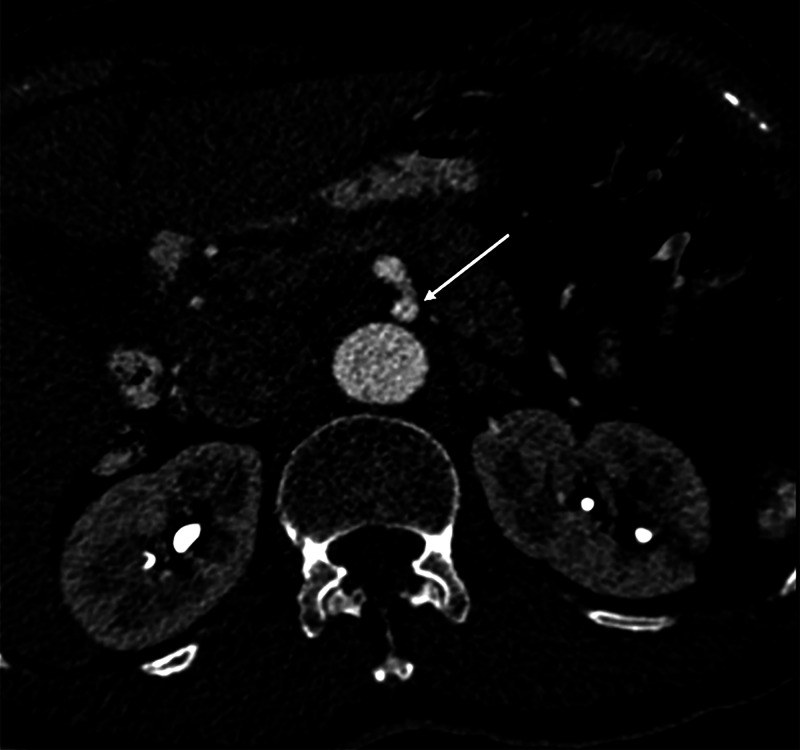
CT aortogram (axial image) demonstrating dissection of CA (white arrow) with patent true and false lumens. CA, celiac artery
